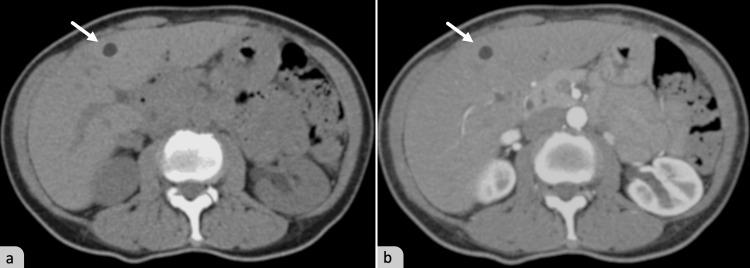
Abdominal axial CT scan showing a hypointense lesion (arrow) in segment IVb of the right liver (a), the lesion does not show enhancement after contrast injection (b).
